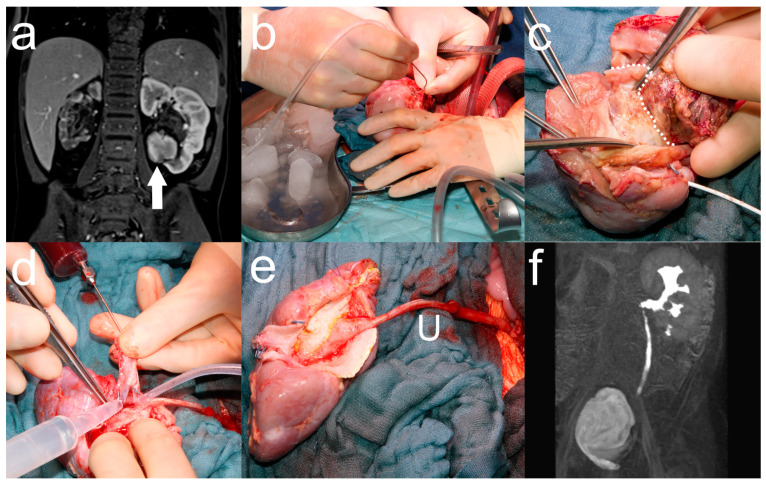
Ante situ tumor resection and orthotopic renal autotransplantation for bilateral nephroblastoma. A 3-year-old boy with bilateral nephroblastoma and dysplastic right kidney and central tumor of the left lower pole (arrow) on preoperative MRI (a). Ante situ mobilization of the left kidney on sterile ice water and rinsing with HTK solution (b). Resection of the central parts of the tumor (above the white dotted line) (c). Blood drawn from the patient being injected into the renal vessels to identify and suture vascular injuries (d). Reconstructed left kidney with ureter (U) after tumor resection (e). Maximum intensity projection (MIP) of postoperative MR urography showing adequate function of the reconstructed left kidney (f).
